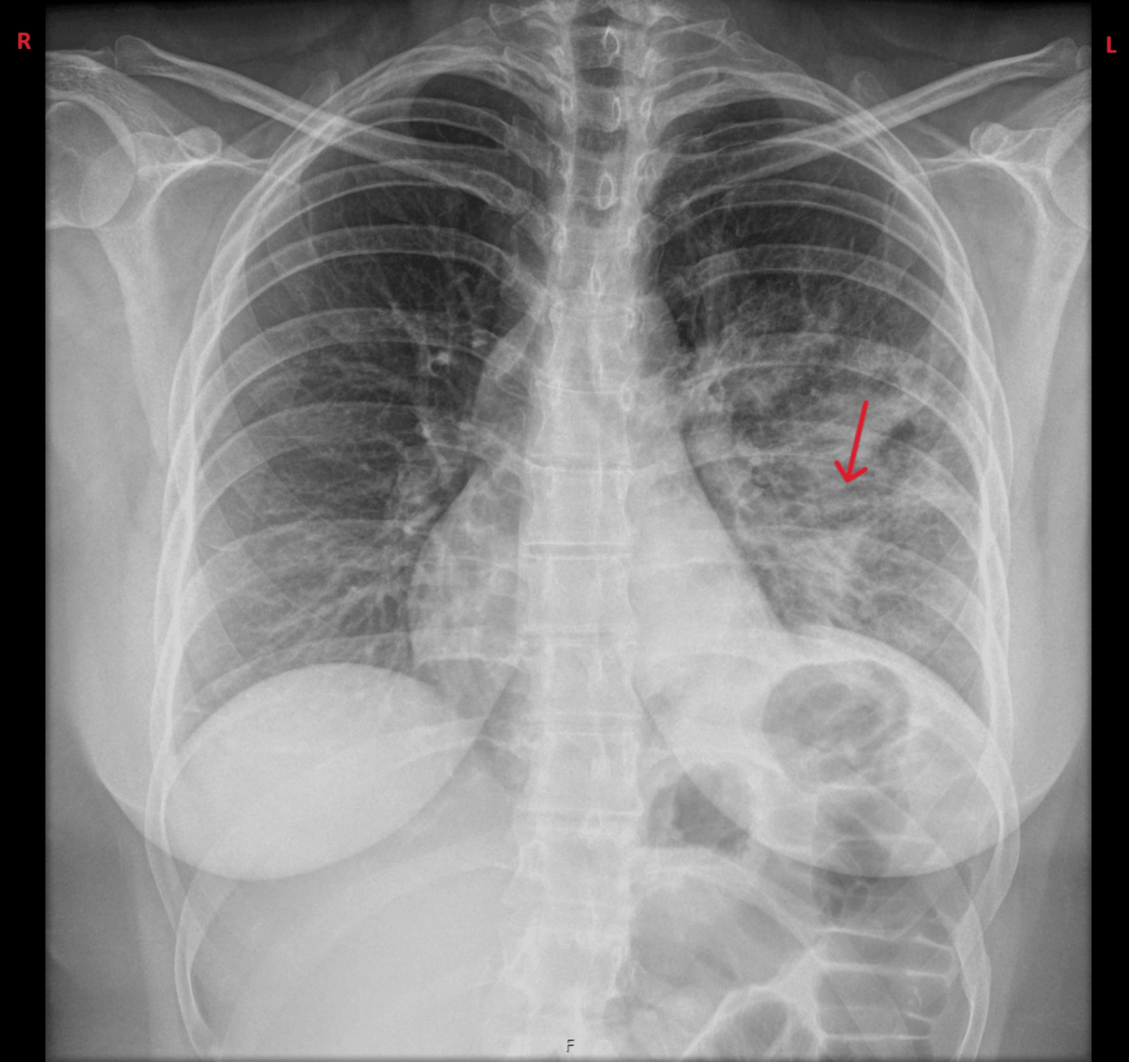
Chest radiograph showing left lower lobe consolidation consistent with pneumonia (red arrow).
